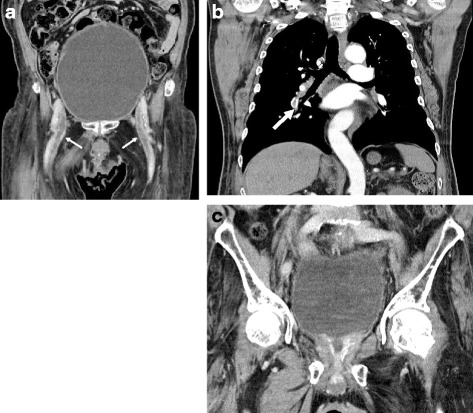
a, b; Contrast-enhanced computed tomographic scan shows thrombi in both the bilateral intrapelvic veins (small arrows) and the right pulmonary artery (large arrow), with an extremely distended bladder. c; Computed tomographic scan shows a postoperative change of transurethral resection of the prostate. The estimated prostate volume was 15 ml, which indicated that the patient did not have benign prostatic hyperplasia
